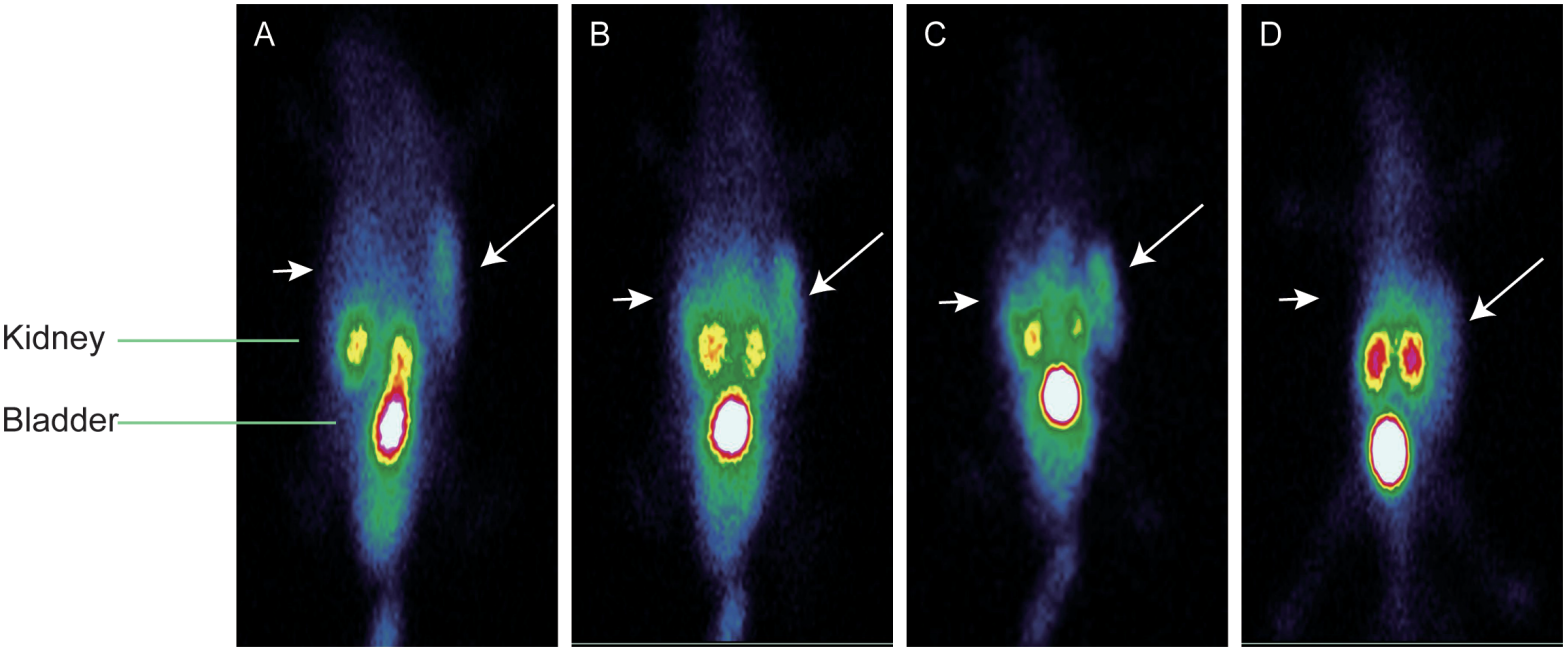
Representative single photon emission–computed tomography maximum-intensity projection images of ^99m^Tc-PDA in dual-flank MC38-B7H1/MC38 xenograft-bearing mie; long arrows indicate MC38-B7H1 tumors, and short arrows indicate MC38 tumors. (A) Images at 30 min. (B) Images at 60 min. (C) Images at 120 min. (D) Blocking images at 60 min.
